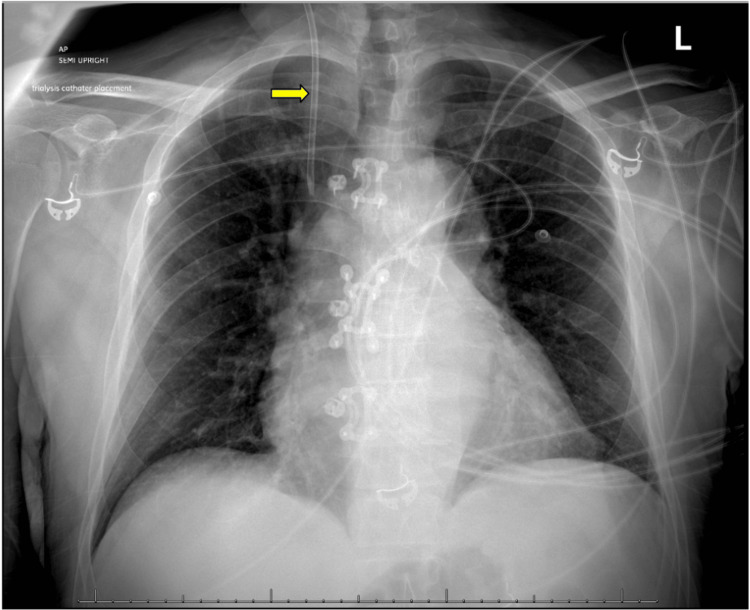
Chest X-ray showing normal heart size without consolidations or pleural effusion and a right internal jugular catheter indicated by the arrow
